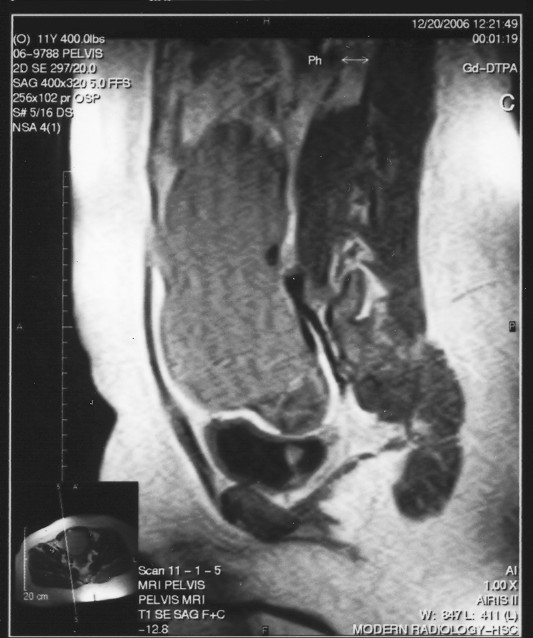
Sagittal T1-weighted MRI. A large, fluid-density, multilobular cystic structure is seen roughly at the midline and extending to the level of the umbilicus. Although the cyst appears to originate on the right, it was discovered at laparotomy to be attached to the left fallopian tube.
